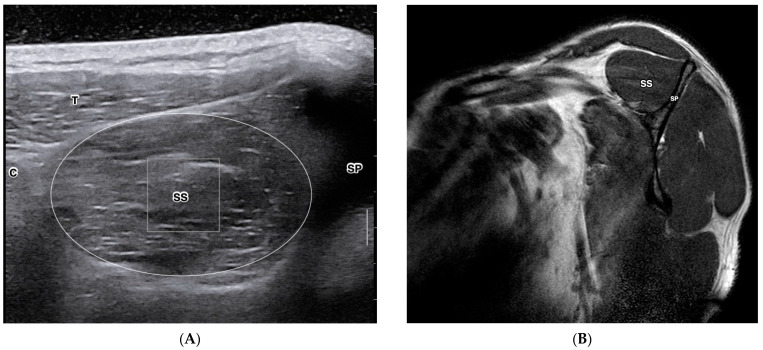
Cross-sectional view of the supraspinatus muscle of the right shoulder in a healthy 29-year-old female. Short-axis view of supraspinatus muscle in B-mode diagnostic ultrasound (A) and MRI T1 sagittal-oblique view (B). C—Clavicle; SP—Scapular Spine; T—Trapezius; SS—Supraspinatus. B. T1W MRI Y-view. SP—Scapular Spine, SS—Supraspinatus.
